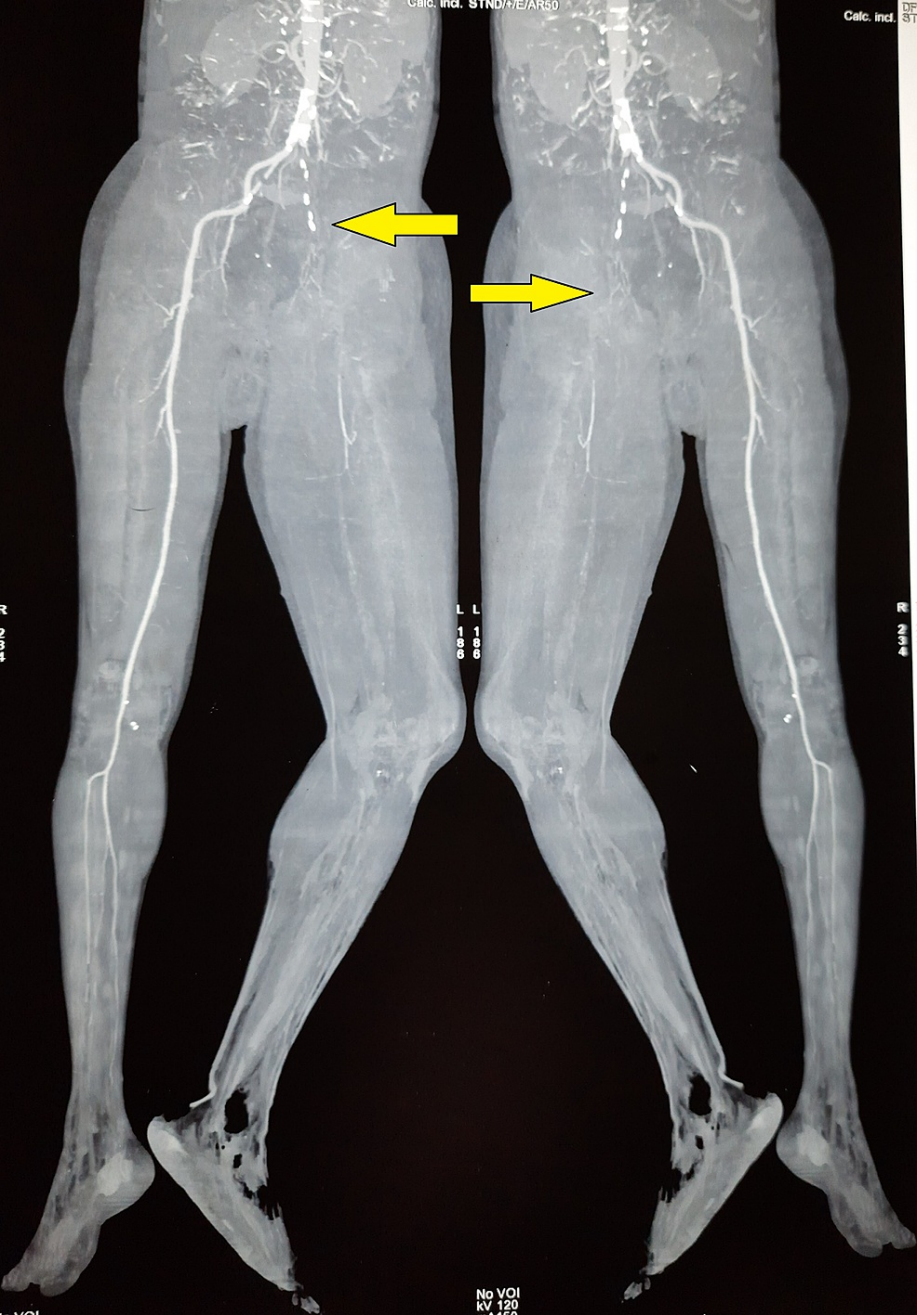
Maximum intensity projections of CTA of bilateral lower limbs showing complete occlusion of the blood supply to the left lower limb from the level of common iliac artery (Arrow). Poor collaterals noted. No distal reformation of the blood flow visualized. Also seen are the cellulitic changes in the left foot with loss of soft tissue. The blood supply to the right lower limb is preserved CTA = CT Angiography
